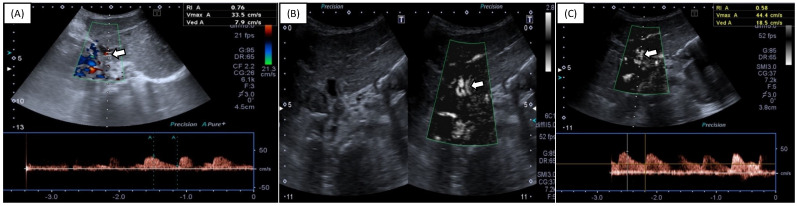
A one-year-old female undergoing abdominal US follow-up for LT, with standard CDI (A) and SMI (B,C). SMI allowed for improved HA visualization and sampling over CDI, with a more definite and stable waveform (C). Arrows indicate HA. HA—hepatic artery; CDI—conventional Doppler imaging; SMI—superb microvascular imaging.
